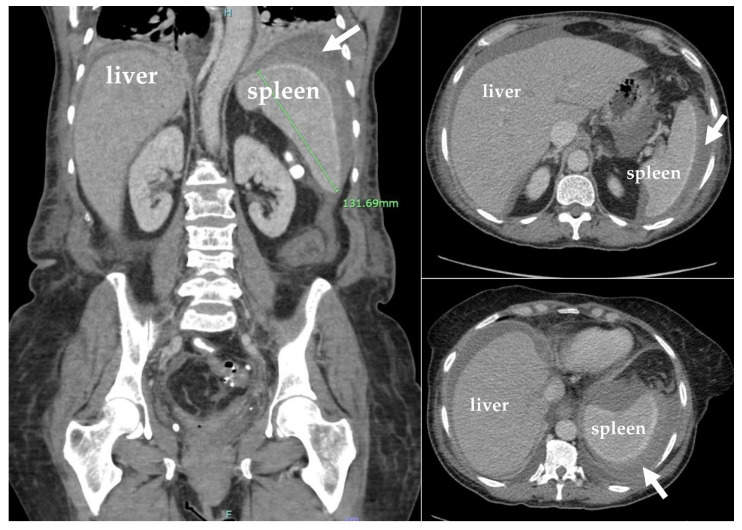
Coronal and sagittal plane computed tomography (CT) scans with IV contrast of the abdomen and pelvis showing hemoperitoneum (white arrows) and mild splenomegaly (13.2 cm).
